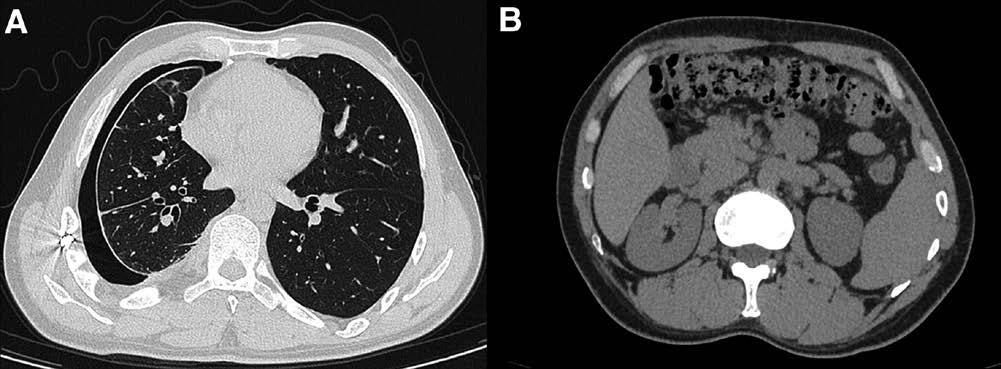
January 17, 2025, CT: (A) scan revealed a small amount of pneumothorax on the right side, (B) no significant pneumoperitoneum were observed in the abdominal cavity. CT = computed tomograph.
